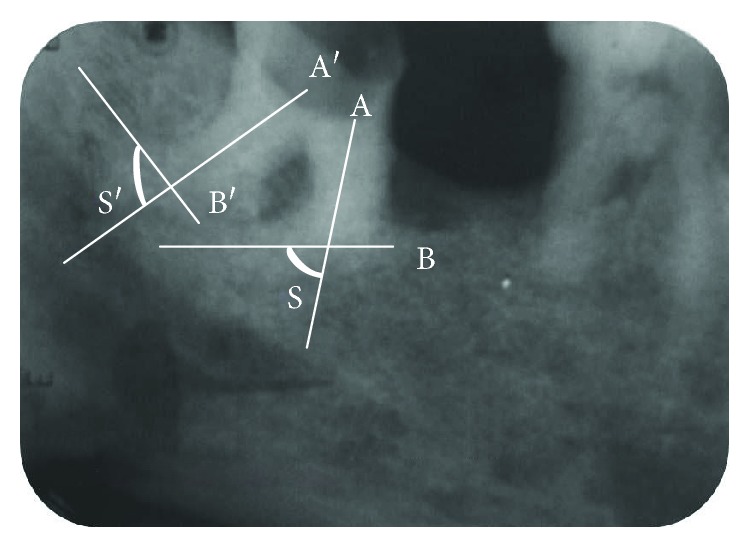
Measurement of Schneider's angle using radiograph. Both *S* and *S*′ are more than 70° (severely curved canals).
